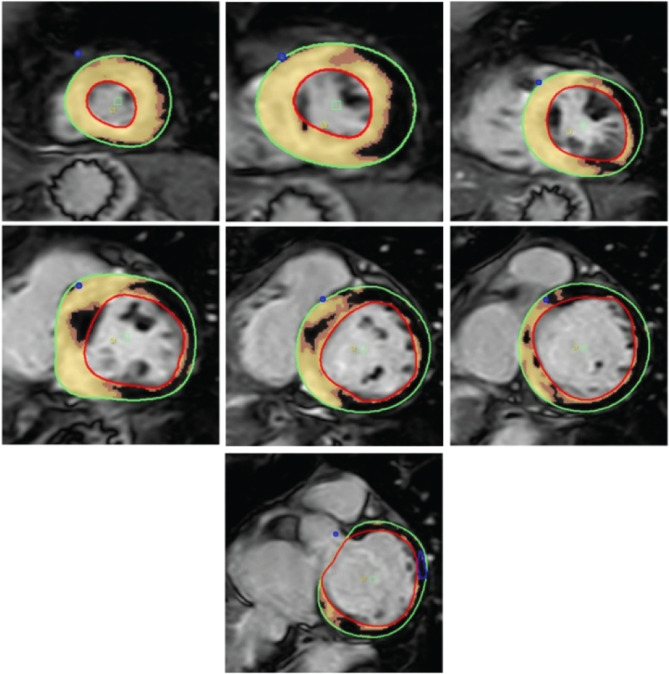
Cardiac magnetic resonance imaging of a patient with a history of hypertrophic cardiomyopathy and VT demonstrating the complexity of the myocardial substrate and the potential need for epicardial ablation. A dense scar with high signal intensity is delineated in yellow, and the scar border zone is delineated in orange.
